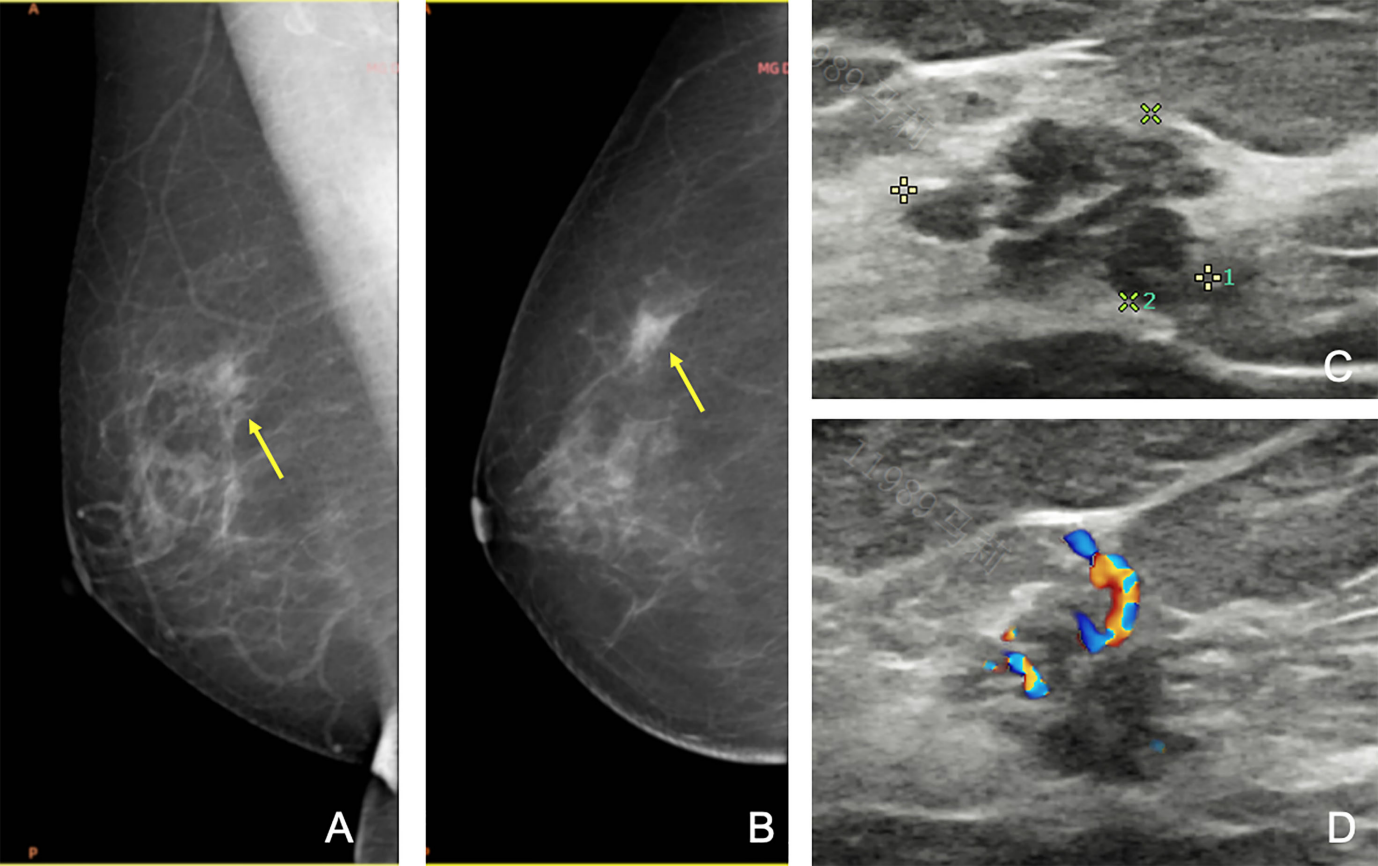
A 69-year-old woman was referred to our hospital for a category 5 mass on the left breast, and the second-look US found a new 4a mass on the right breast, which was missed at the initial assessment. Craniocaudal (A) and mediolateral oblique (B) mammograms of the right breast showing focal asymmetric dense tissue (arrows). However, since the first ultrasound failed to find any lesions, this lesion was unreported. (C, D) Second US showing a heterogenous lesion with micro-lobulated margin and linear vascularity. After reviewing the mammography, this lesion was re-categorized as 4a. Subsequent biopsy confirmed ductal *in situ* carcinoma. The patient finally received breast conservative surgery on both sides.
